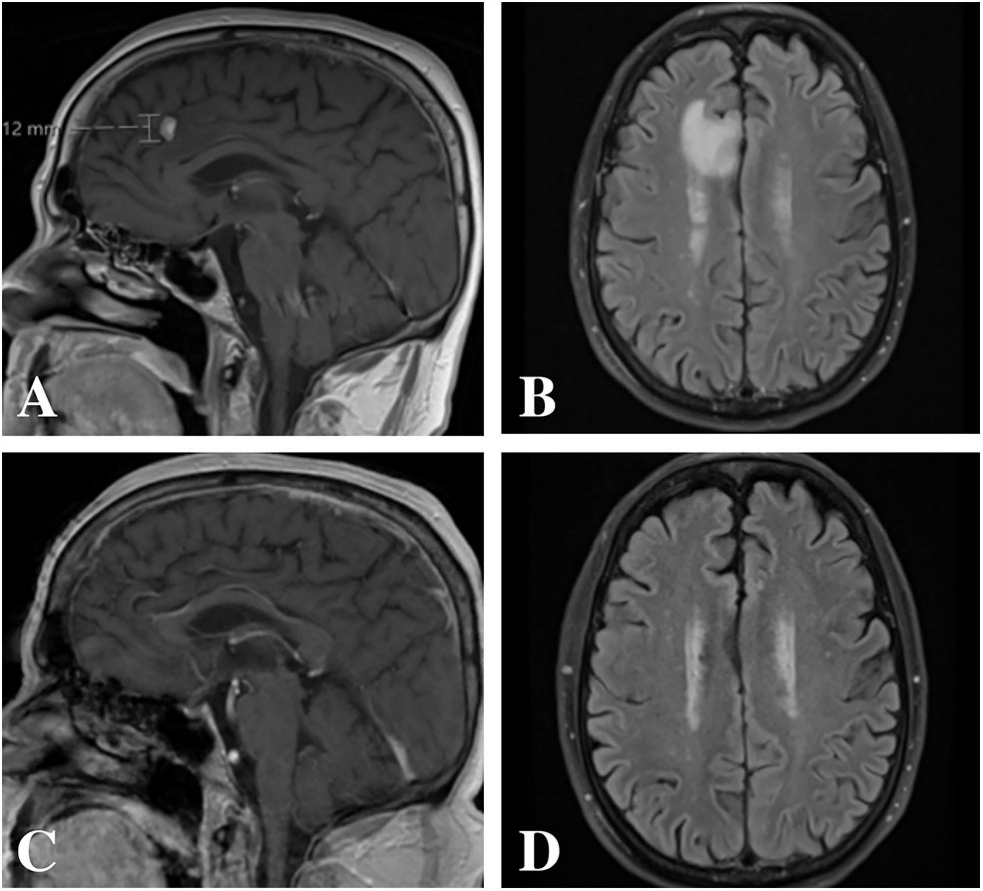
Magnetic resonance imaging pre- and post-treatment. (A,B) Pre-treatment imaging demonstrated an avidly enhancing 11 × 7 × 12 mm lesion along the mesial surface of the right frontal lobe within the cingulate sulcus with surrounding vasogenic edema. (C,D) Post-treatment imaging with complete radiographic resolution of the lesion and associated vasogenic edema.
